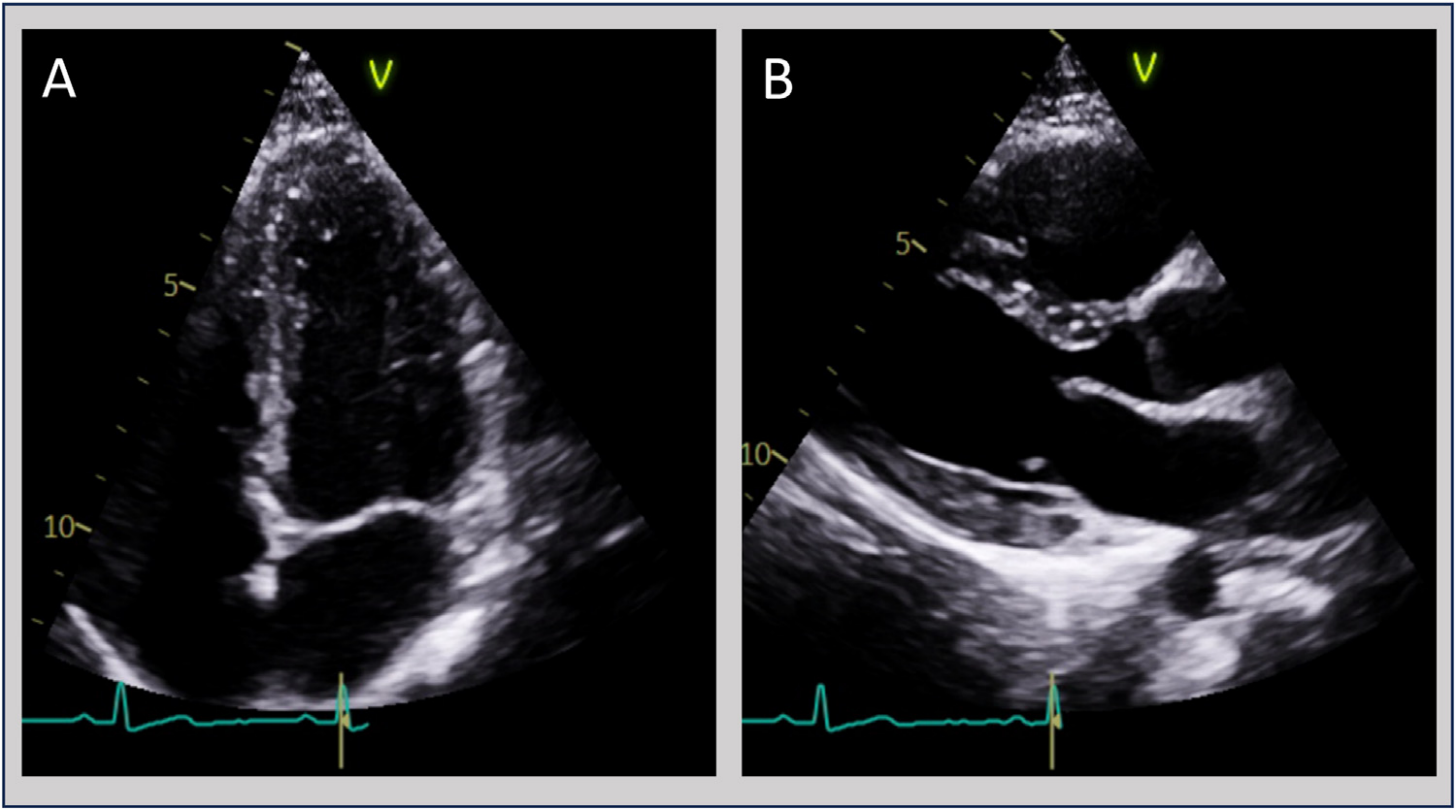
Echocardiogram images showing normal left ventricular cavity dimensions and wall thickness with no pericardial effusion observed. Image (A) showing the apical four-chamber view. Image (B) showing the parasternal long axis view.
